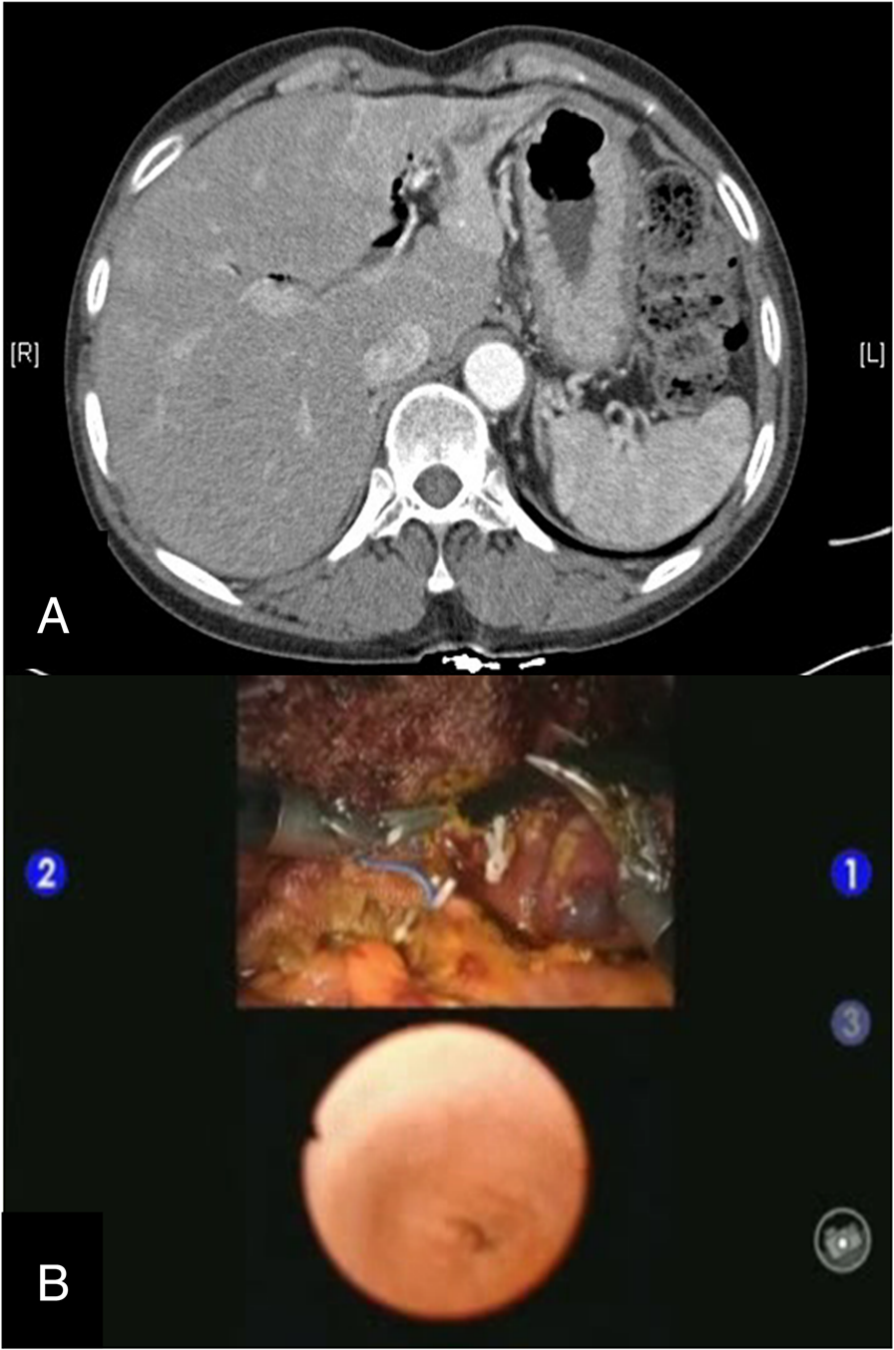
Images from a preoperative abdominal CT scan and multi-input view of intraoperative common bile duct exploration. A preoperative CT scan of the patient with cholangiohepatitis shows intrahepatic ductal dilatation and left lobe atrophy (A). Intraoperative robot-assisted common bile duct exploration was facilitated by simultaneous visualization of the images from the choledochoscopy on the surgeon’s console (B).
